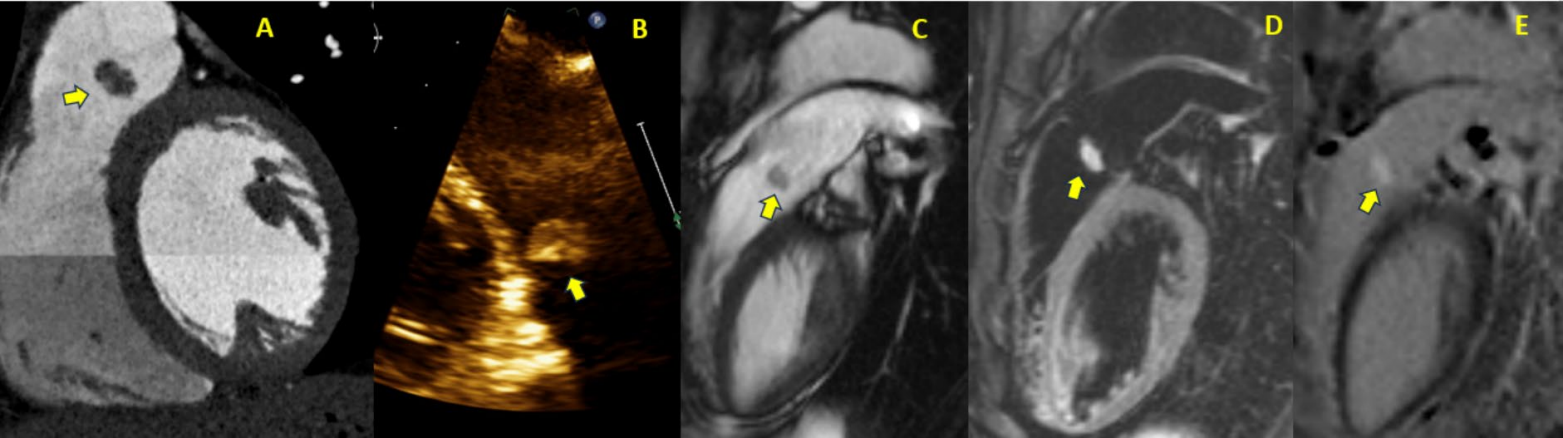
42-year-old female presented with chest pain. CT coronary angiogram, performed to rule out coronary artery disease, incidentally revealed a rounded mass attached to her pulmonic valve leaflet (*Image A*). This finding was confirmed on transthoracic echocardiogram (RVOT view) (*Image B*). The differential diagnosis included a benign tumor or a vegetation, although endocarditis was thought to be less likely as the patient did not have any signs or symptoms of systemic infection. For further tissue characterization, the patient underwent cardiac MRI. SSFP bright blood imaging (*Image C*) showed the mass attached to the pulmonic valve. Edema-weighted imaging (STIR) (*Image D*) revealed increased signal intensity of the pulmonic valve mass, indicating increased water content. Late gadolinium-enhancement imaging (*Image E*) demonstrated diffuse gadolinium uptake within the mass, suggesting a fibroblastoma. This case highlights the tissue characterization capabilities of cardiac MRI using multiple sequences and emphasizes the ability of MRI to differentiate vegetation from a benign tumor, particularly when the echodensity has limited mobility. The patient opted for surgical removal of the mass, which was confirmed to be a pulmonary valve fibroblastoma.
